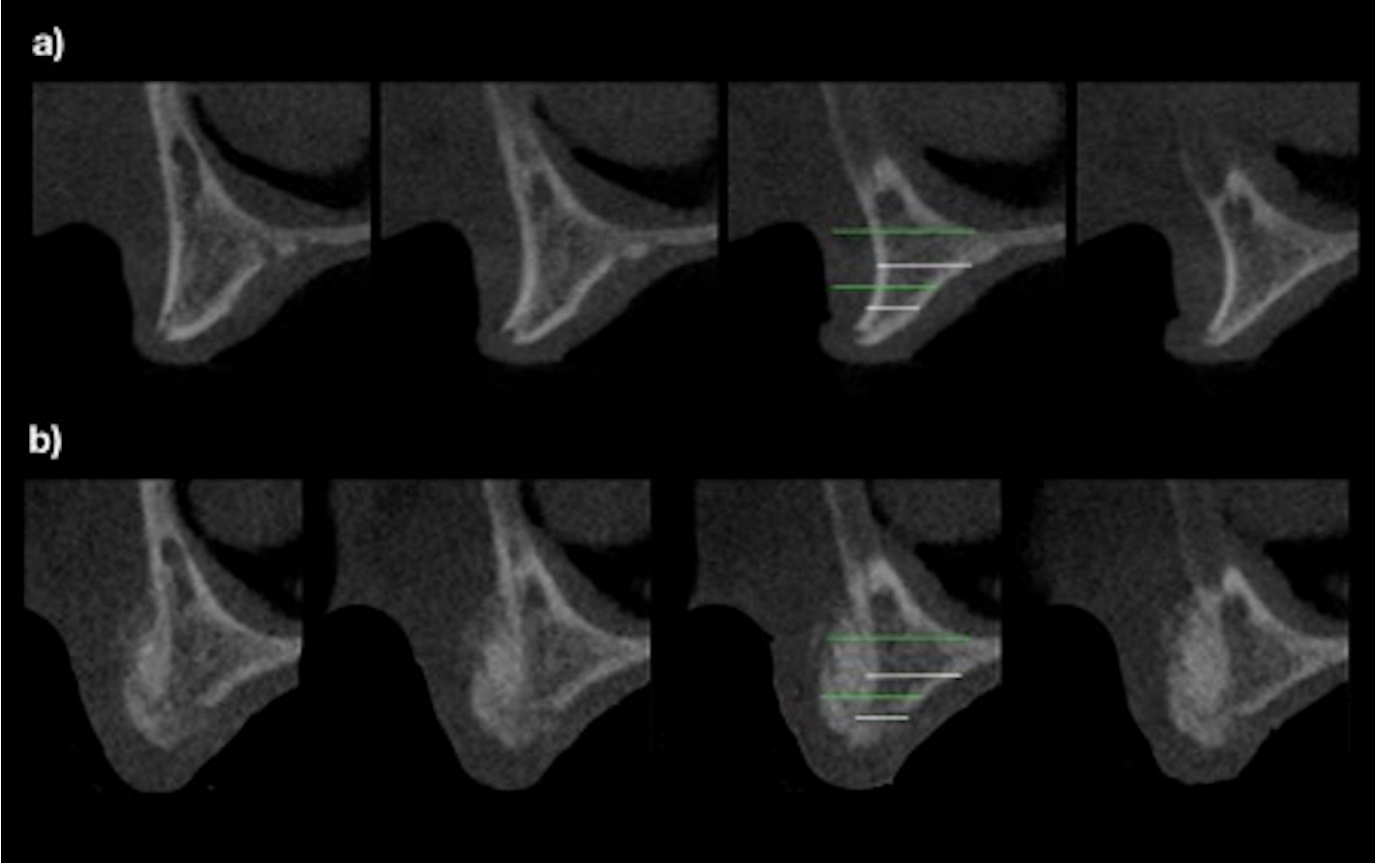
White line showing the measurement in the CBCT before bone graft (a) and green line showing the measurement in the CBCT before implant placement (b) superimposed on both tomographies to facilitate visualization of the bone gain.
